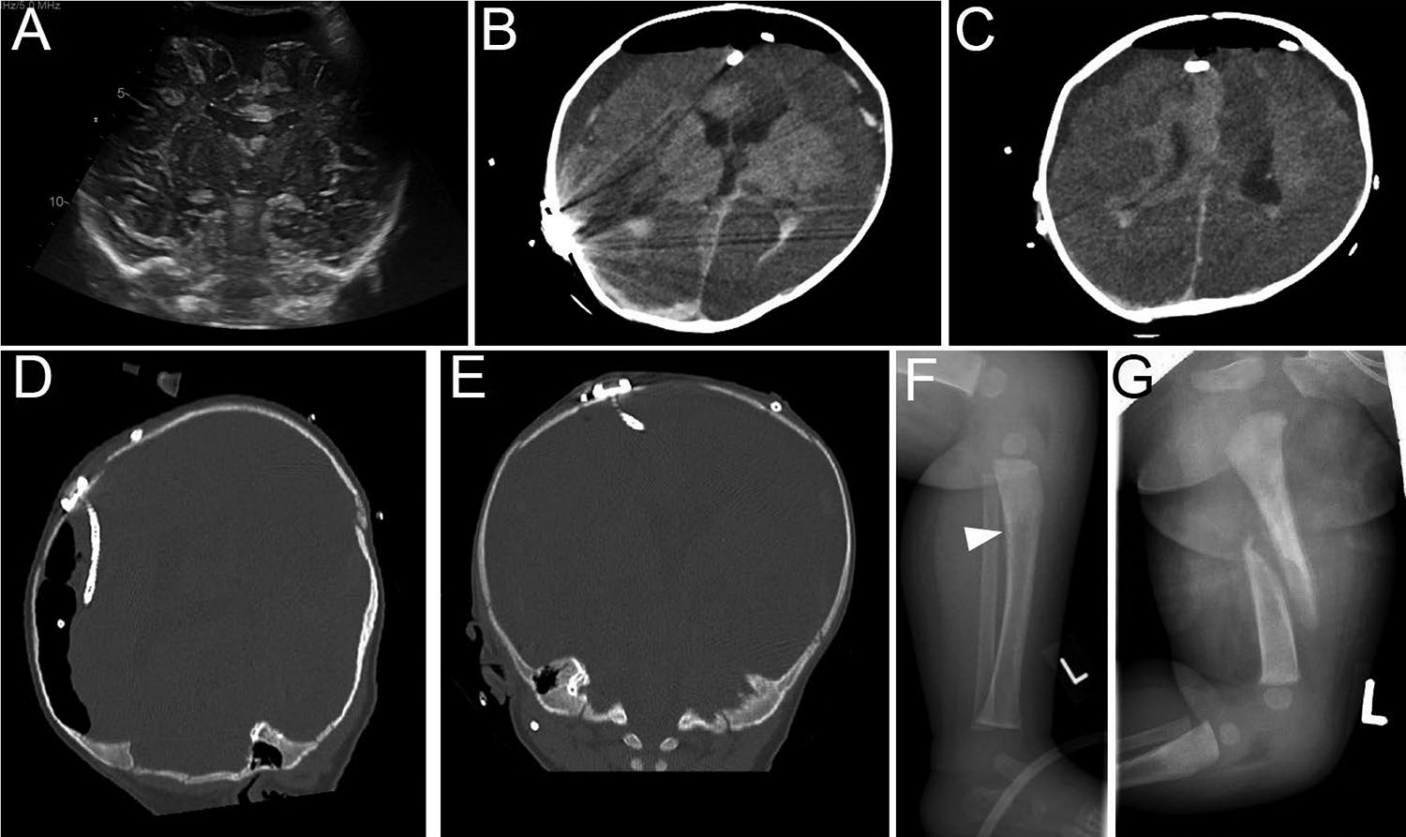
The imaging in the further patients’ course after he was readmitted because of massive clinical deterioration in postictal unconscious state. A The cranial ultrasound on admission was rather unspecific, but since clinical signs indicated massively raised intracranial pressure, the reservoir was tapped and high ICP was confirmed. The patient was directly transferred to the OR. The immediate postoperative CT scan was indicating bilateral extensive cortical infarction as hypodensity changes (B, C). The dislocation of the ventricular catheter on the right side (D, E) as well as further workup with X-rays identifying fractures of the left tibia in (F) and the left femur (G) proving severe abusive traumatic injuries
